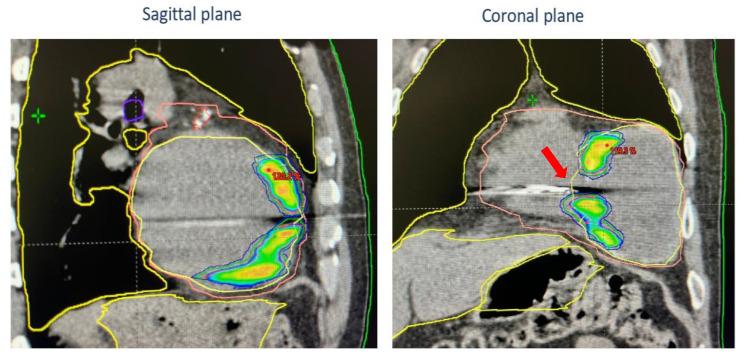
Dose distribution and treatment plan shown in sagittal CT images (left) and coronal plan (right). The areas outlined by the yellow, violet and red lines correspond to the organs at risk (OARs). The area outlined by the pink area corresponds to the heart volume. Colored areas correspond to isodose level of RTplan. Notice that the ICD lead was excluded from treatment (red arrow).
